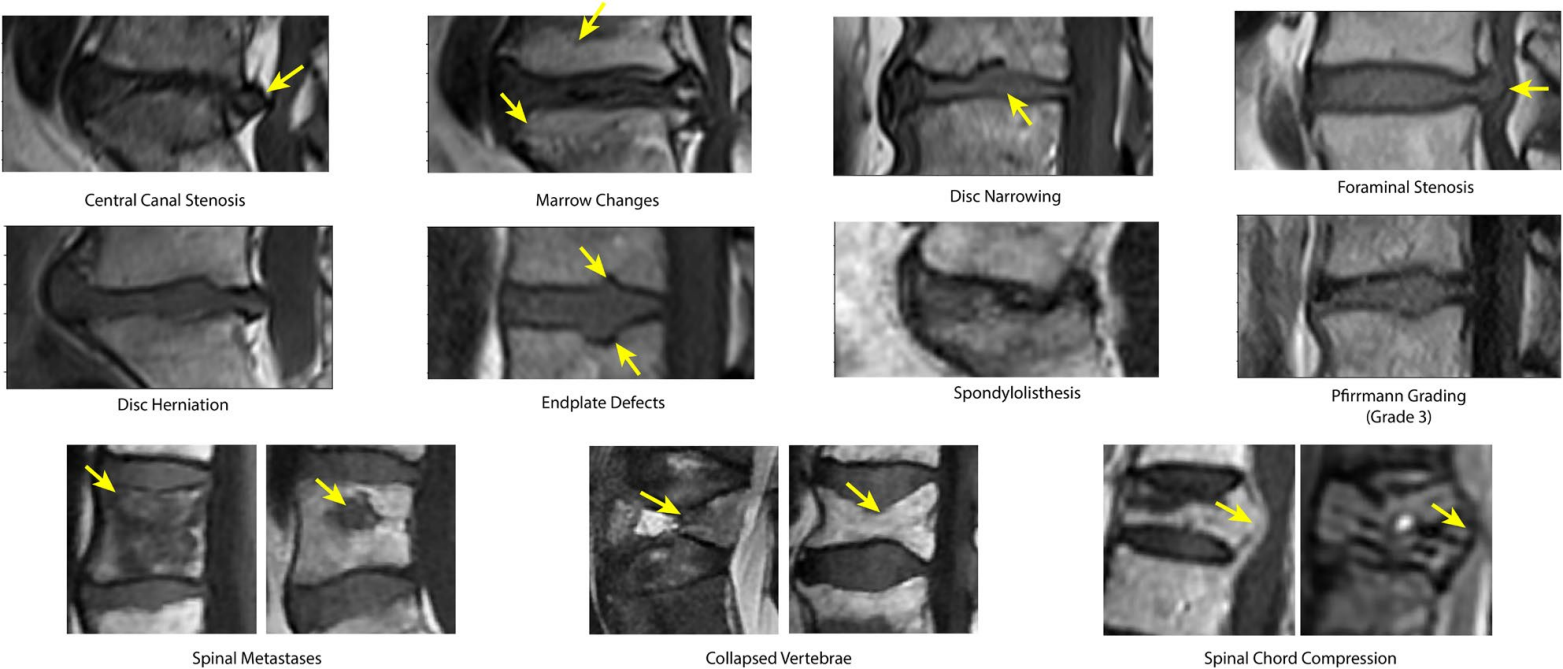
Spinal MR imaging analysis tasks considered in this paper. The top two rows show degenerative changes, graded for each intervertebral disc in the scan. The bottom row shows tasks related to spinal cancer, where predictions are made for each detected VB.
